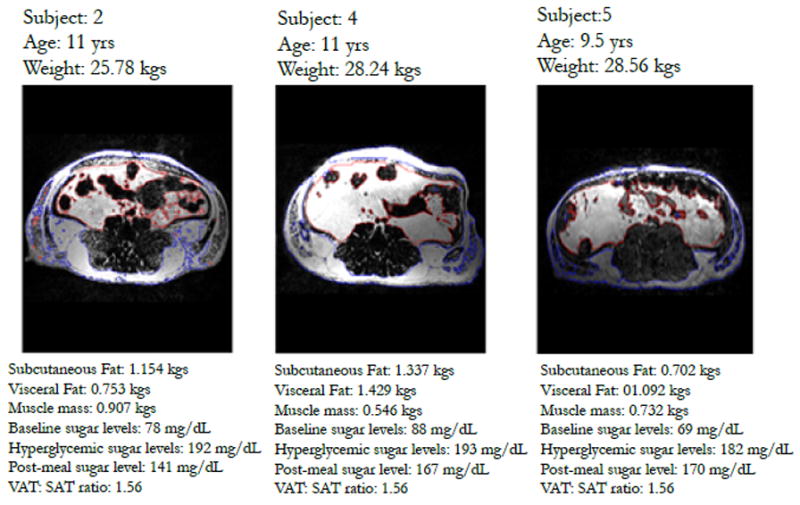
Three representative MRI abdominal fat images of baboon subjects. Note the increase in visceral fat, decrease in subcutaneous fat ratio across subjects and an increase in fasting glucose. (Blue region) subcutaneous fat and (Red region) visceral fat region.
